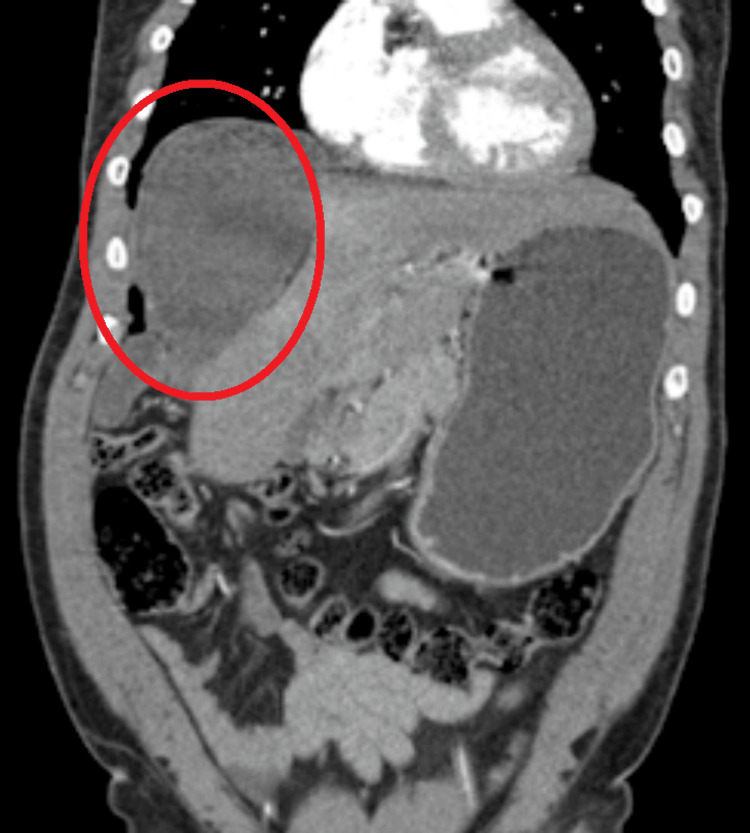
Initial CT angiography with contrast, coronal view. There is a well-circumscribed, rounded structure in the right upper quadrant (circled in red) with predominantly fluid attenuation. A large, well-circumscribed, low-attenuation cystic lesion occupying the left upper quadrant of the abdomen, likely arising from or abutting the stomach. The lesion demonstrates homogeneous fluid attenuation without internal septations, mural nodularity, or obvious solid enhancing components. There is significant mass effect with displacement of adjacent structures, including inferior displacement of bowel loops and medial deviation of surrounding viscera. The stomach appears compressed and displaced, suggesting either an exophytic gastric origin or close association with the gastric wall. No obvious pneumoperitoneum. No free fluid is definitively identified on this image. The visualized bowel loops are non-dilated without clear obstruction.
